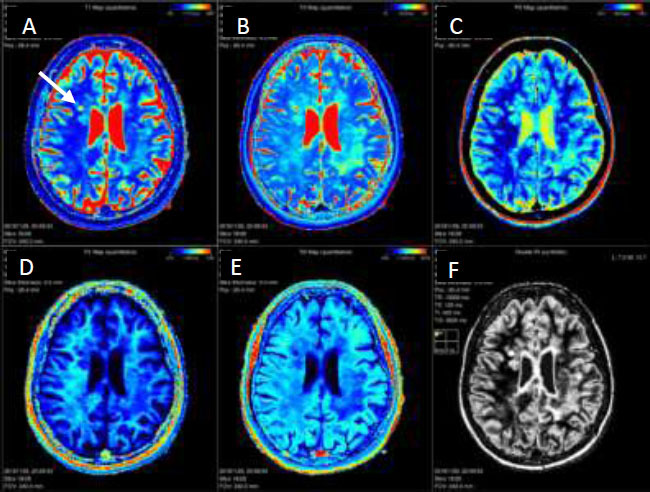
The registration of the images in Fig. (2a) with the corresponding quantitative MRI maps, (A) Axial T1 map, (B) Axial T2 map, (C) Axial PD map, (D) Axial R1 map, (E) Axial R2 map and (F) Synthetic double inversion recovery (DIR) image. The white arrow points to the enhancing MS lesion.
